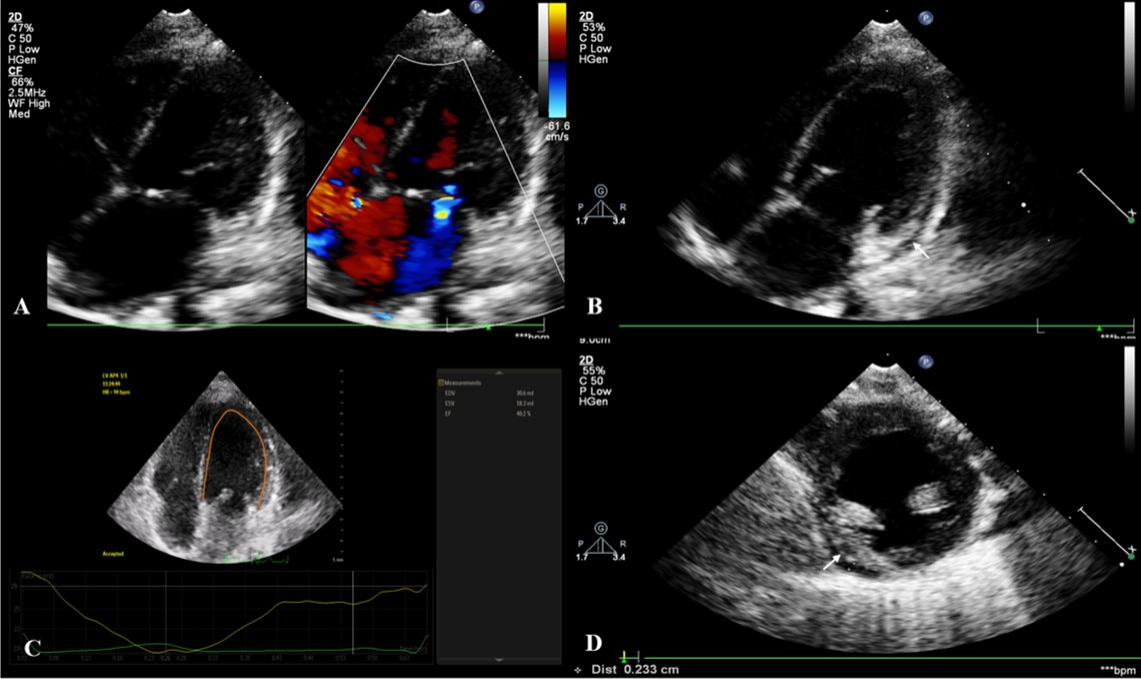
bidimensional and color Doppler echocardiography, apical four chamber view, showing dilated left cavities and mitral regurgitation; B, D: bidimensional echocardiography, four chamber and short-axis views, showing minimal pericardial effusion (arrows); C: left ventricle ejection fraction.
